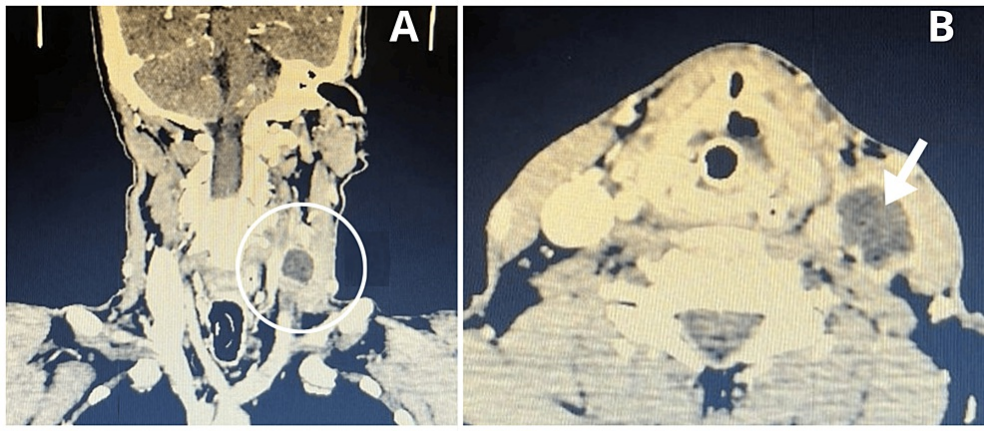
Head CT CT images with contrast in (A) coronal and (B) axial reconstructions demonstrate a hypodense lesion located in the internal jugular vein (white circle), characterized by well-defined borders, an oval-shaped morphology, and a hyperdense periphery (white arrow) suggestive of luminal thrombus occupation in the left internal jugular vein.
